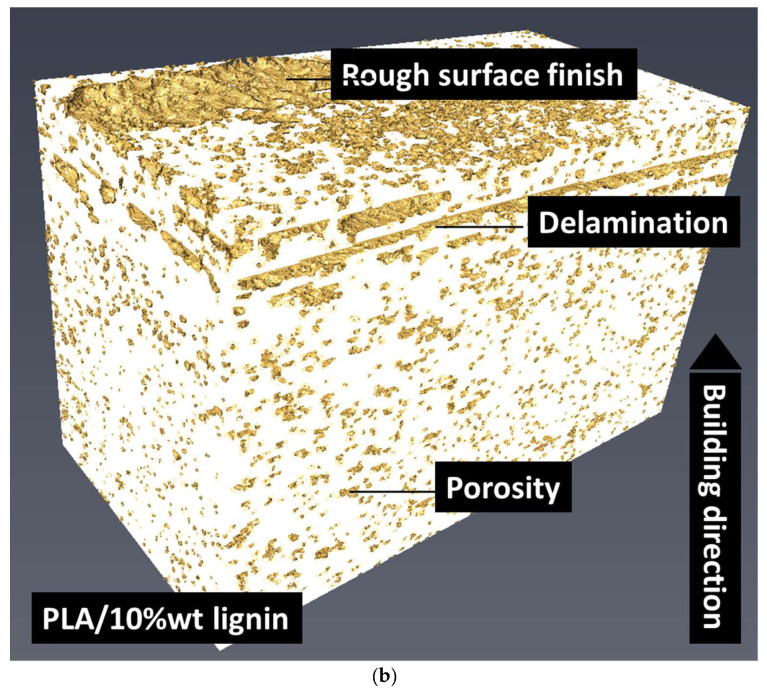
X-ray microtomography results: (a) orthogonal views underlying the microstructure of 3D-printed cured acrylate-modified PLA/10 wt% lignin, (b) 3D view of the porous structure.
